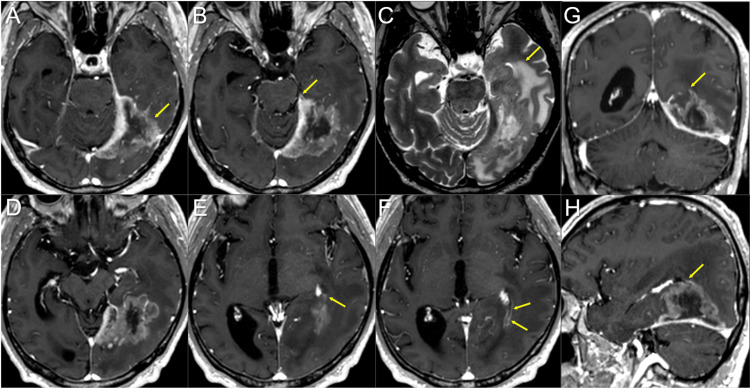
Magnetic resonance images before the upfront 15-fraction stereotactic radiosurgery for the brain metastasis The images show (A, B, D-H) contrast-enhanced T1-WIs, (C) a T2-WI, (A-F) axial images, (G) a coronal image and (H) a sagittal image. (A, B, D-H) These images are shown at the same magnification and coordinates under co-registration and fusions (B, C, the same cross-section; A, D-F, different cross-sections). A heterogeneously enhanced, indistinctly demarcated lesion (arrow in A) with a 58-mm maximum diameter is observed in the left temporo-occipital lobes, and functionally it involves the optic radiation. The mass attaches extensively to the markedly enhanced tentorium cerebelli that thickens toward the tentorial incisura. The extension of the lesion is proximal to the pons at the most ventral side of tentorial incisura (arrow in B). The lesion also infiltrates the trigone of the left lateral ventricle (arrows in E, G, H), and part of the ventricular wall is enhanced (arrows in F). (C) On the T2-WI, most of the lesion shows moderate high-intensity solid components with the unclear boundary with part of the brain parenchyma without edema. The lesion contains internal necrotic high-intensity components, along with the surrounding high-intensity parenchymal edema (arrow in C). WI: weighted image
